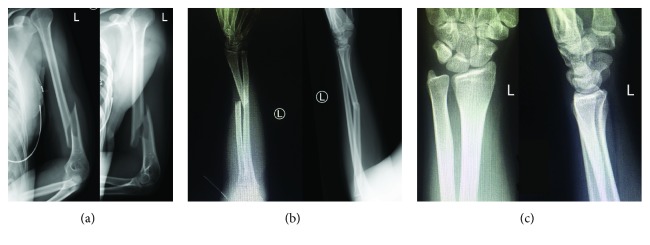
Anteroposterior (AP) and lateral radiographs of the humerus (a), forearm (b), and wrist (c) demonstrating a displaced left distal third humeral shaft fracture, a displaced left midshaft radius fracture with dislocation of the left distal radioulnar joint.
